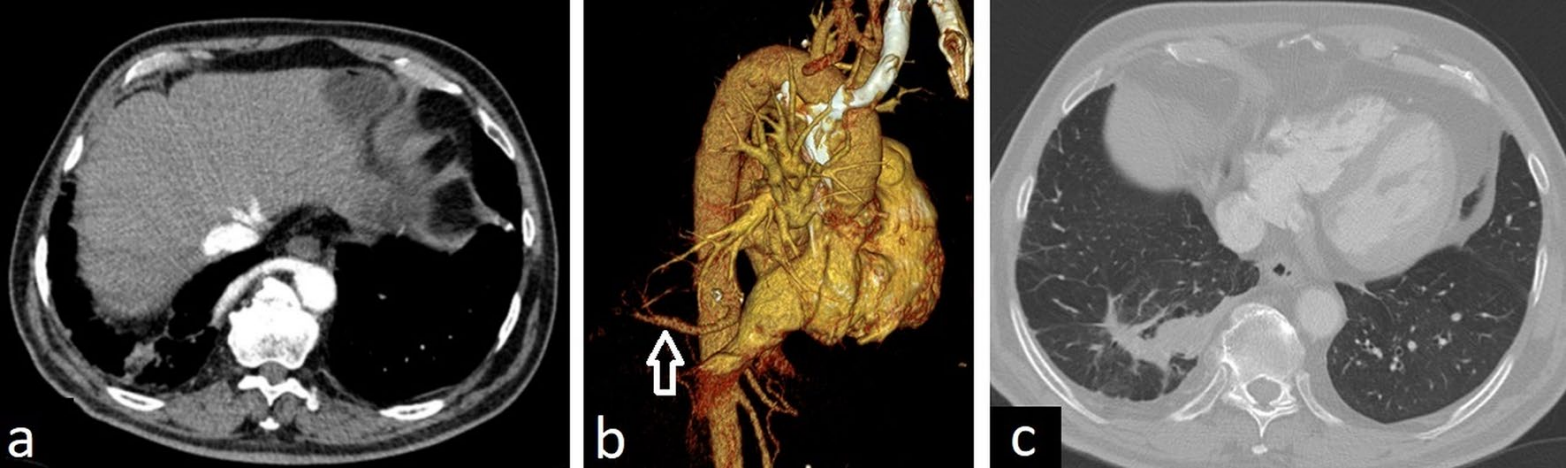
A 67-year-old asymptomatic male with sequestration. Contrast-enhanced axial CT image in the mediastinal window (a) and 3D volume-rendered reconstruction image (b) demonstrate the aberrant artery originating from the descending thoracic aorta (arrows). Axial CT image in the lung window (c) depicts right lower lobe opacity corresponding to the sequestration
